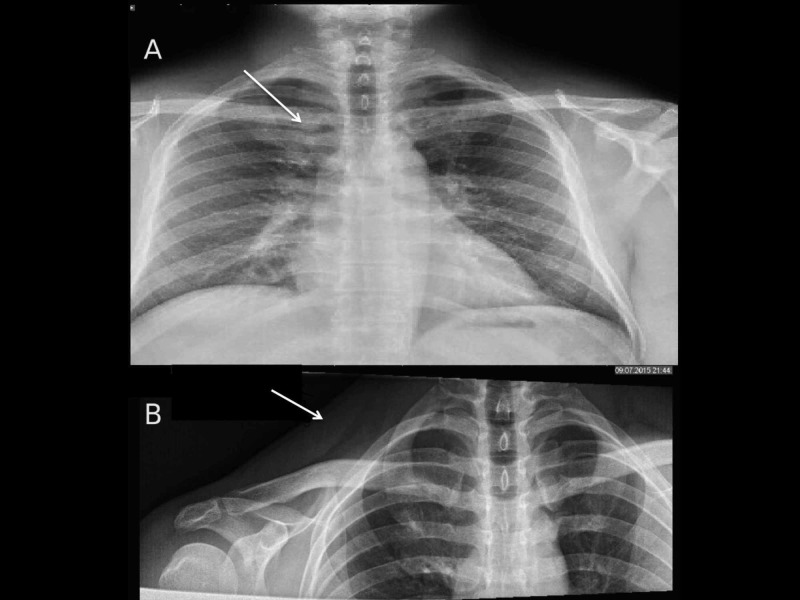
Chest X-ray AP view and right clavicle X-ray AP view. A: Chest X-ray AP view showing a dense opacity in the right para-tracheal area (arrow). B: Right clavicle X-ray AP view showing soft tissue inflammation on the right side of the base of the neck (arrow), but no evidence of fracture or dislocation of the clavicle bone. AP, anteroposterior
